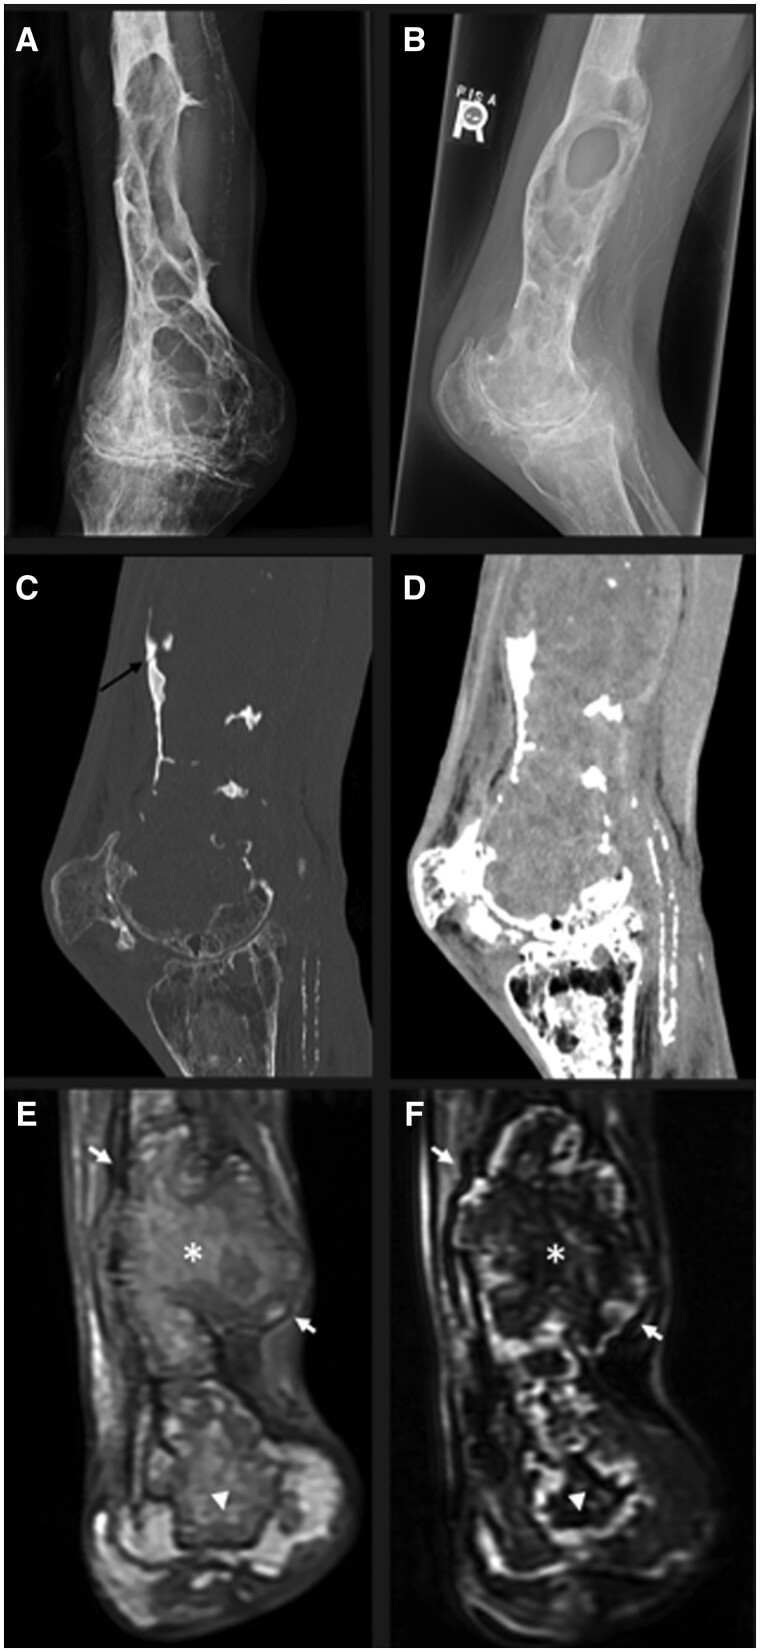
(A) Frontal and (B) lateral radiographs of the knee shows a large lobulated lytic lesion with well-defined margins in the distal femur. Also seen is severe tricompartmental knee arthropathy representing haemophiliac arthropathy. CT in (C) bone and (D) soft-tissue windows shows the extent of osseous destruction secondary to the large soft tissue mass, and the pathologic fracture that was radiographically occult (black arrow). (E) T1 fat-saturation and (F) T2 Short-Tau Inversion-Recovery MRI images in coronal planes showing the mass to be of heterogeneous signal, with regions of high signal intensity on T1-weighted image, and low signal intensity on T2-weighted image representing intracellular methaemoglobin (*), and regions of low T1, high T2 signal intensity representing extracellular methaemoglobin (arrow head). The mass is encapsulated by a well-defined hypointense fibrous capsule (white arrows).
